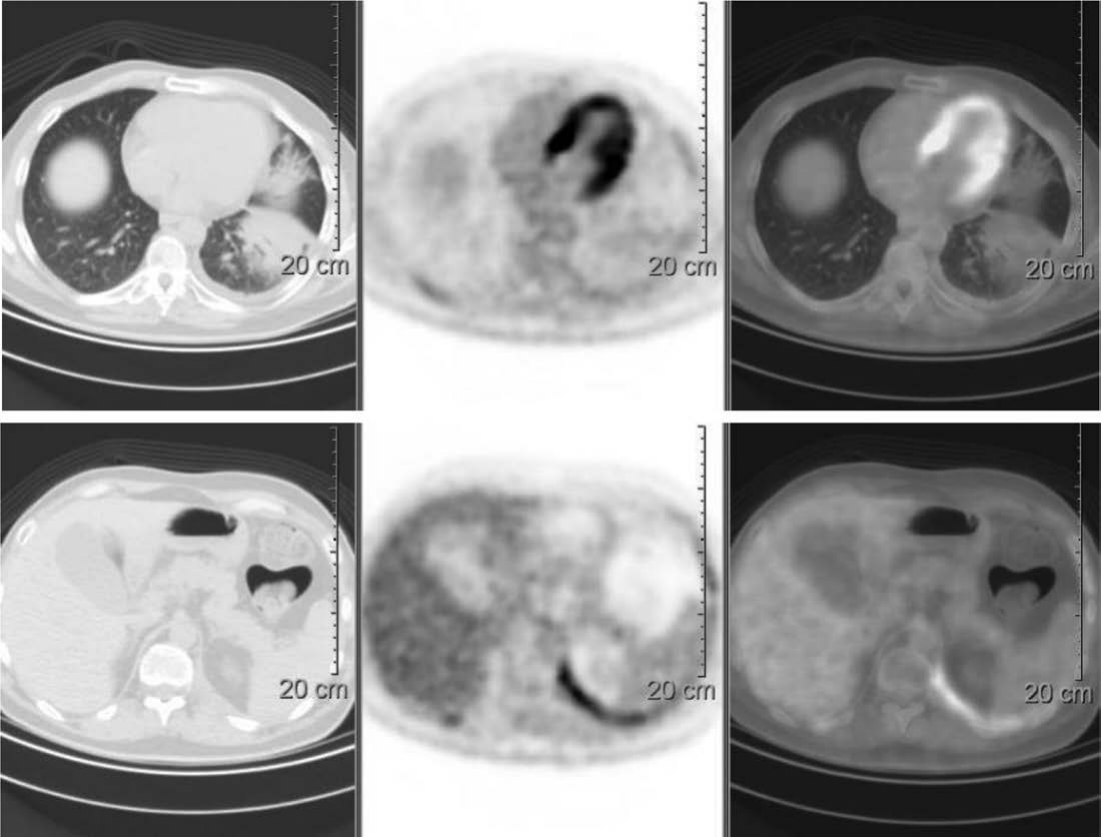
18F-FDG PET: preoperative mild diffuse tracer uptake in multiple areas of the left pleura.
